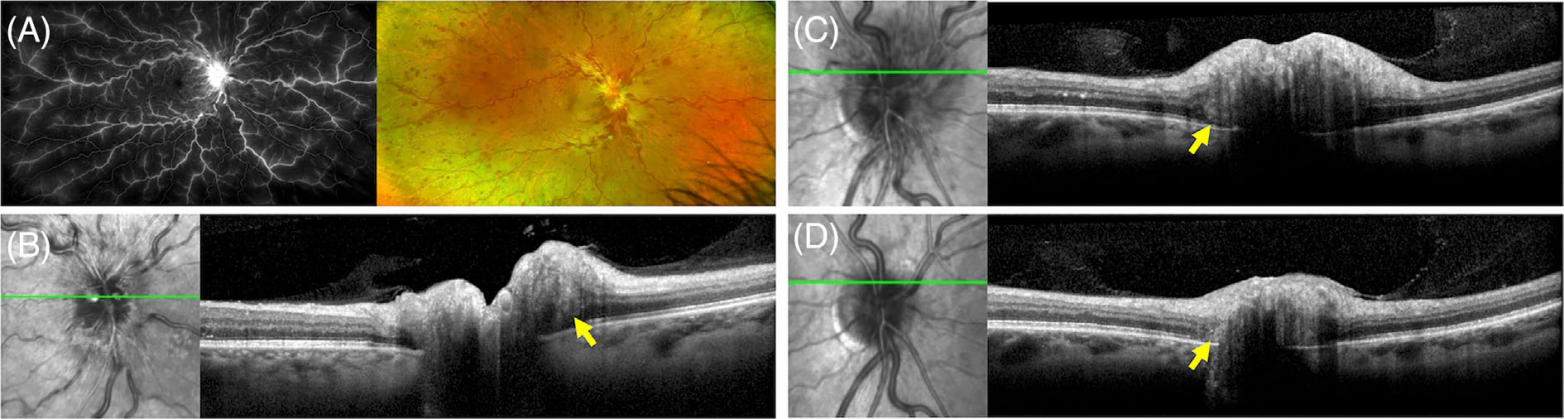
A right nonischaemic central retinal vein occlusion in a 53‐year‐old female (A) with accompanying disc oedema. OCT through the disc showed peripapillary hyperreflective ovoid mass‐like structures (PHOMS) nasally as marked by yellow arrows (B). A 42‐year‐old male with type 2 diabetes mellitus presented with right disc oedema with no visual loss and normal magnetic resonance imaging (MRI) (C). The presumed diabetic papillopathy resolved spontaneously 12 months later with partial regression of PHOMS as marked by the yellow arrows (D).
